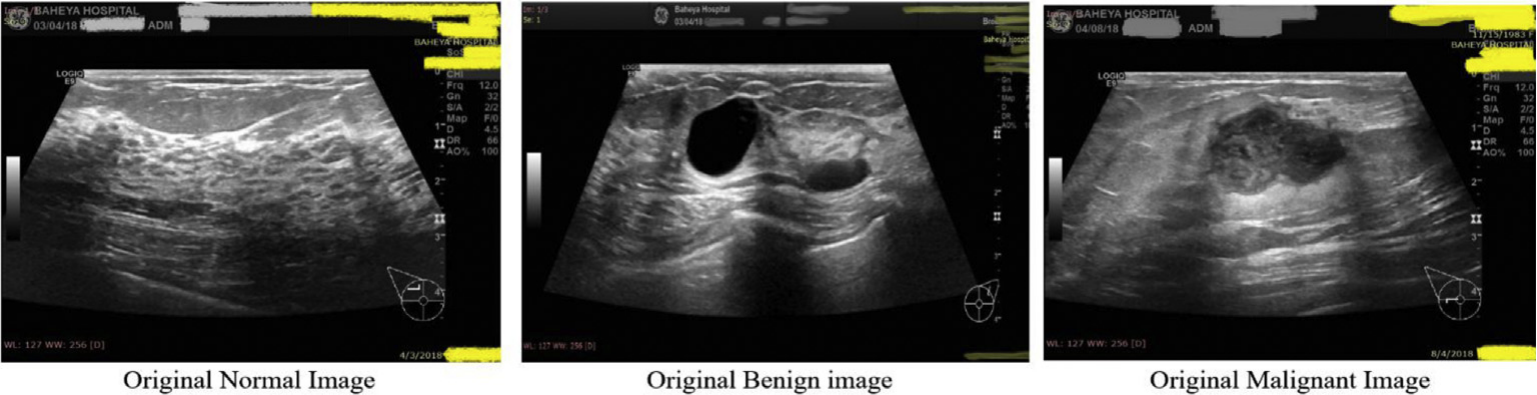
Samples of original Ultrasound breast images dataset (Original images that are scanned by the LOGIQ E9 ultrasound system).
